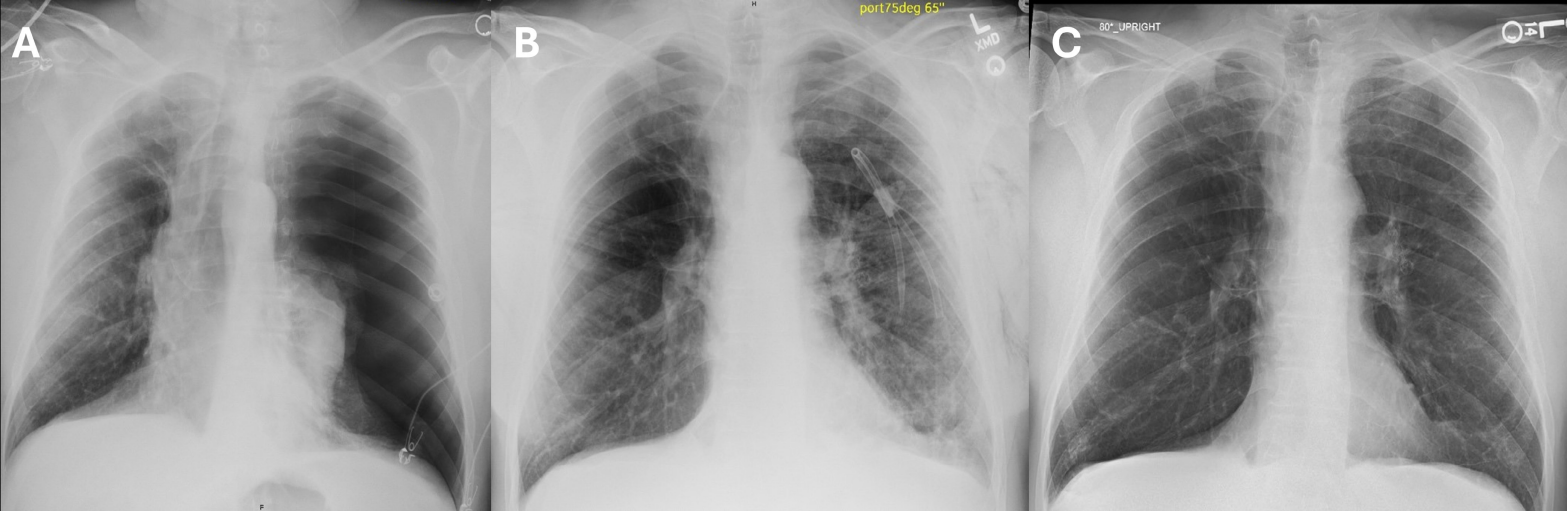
Case #2 patient radiographs. (A) Large left sided pneumothorax post single‐stage BLVR. (B) Persistent air leak after two valves removed, left tube thoracostomy allowed for lung expansion. (C) No pneumothorax after staged BLVR, tube thoracostomy removed.
